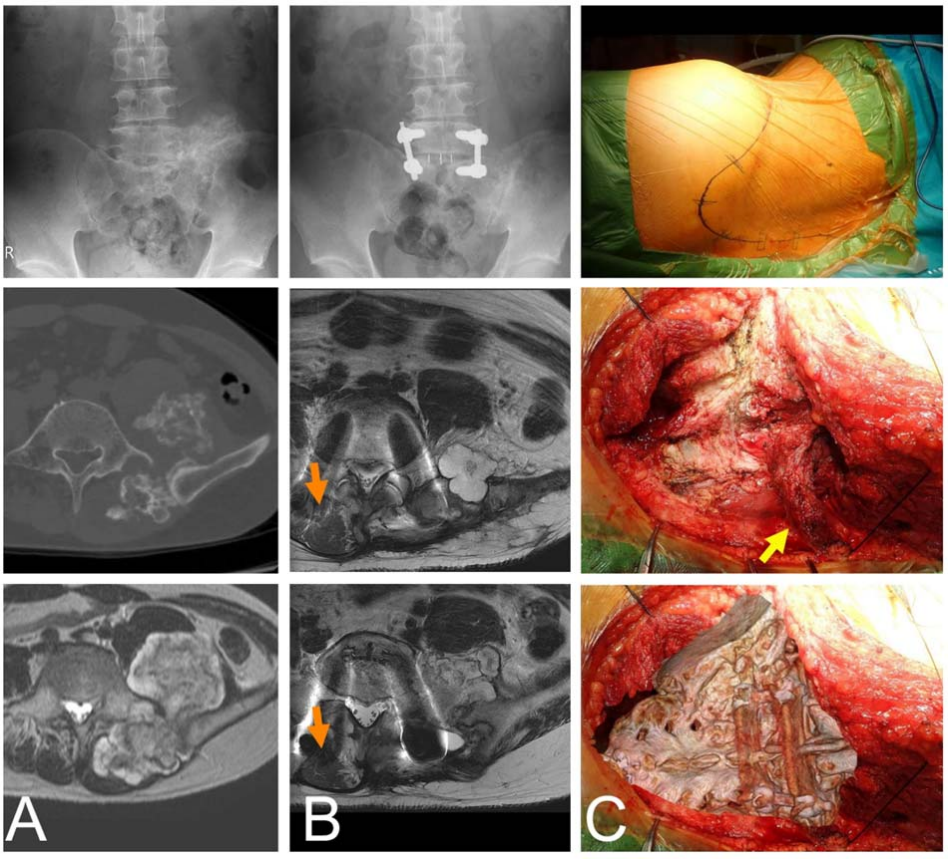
Chondrosarcoma in the sacrum of a 44-year-old male. A plain radiograph (A, top) and CT (A, middle) shows an osseous lesion in the sacrum. The osseous lesion is covered by a cartilaginous lesion with a high signal T2 weighted image (A, bottom). L5 and S1 were fused with screws in the initial operation (B, top). Eleven months after the initial operation cartilaginous nodules appear as a recurrent lesion at L5 (B, middle) and S1 (B, bottom). The once elevated distal multifidus muscle on the non-tumor side was morphologically almost normal (orange arrows), but the distal multifidus muscle on the tumor side was still thin. The patient was in the right-side bottom decubitus position. The skin marker shows the convex skin incision at the distal sacrum posteriorly (C, top). The sacrum is viewed by retraction of the multifidus muscle (yellow arrow) (C, middle). The image of the sacrum is overlapped on the C-middle picture to show the anatomical location (C, bottom).
